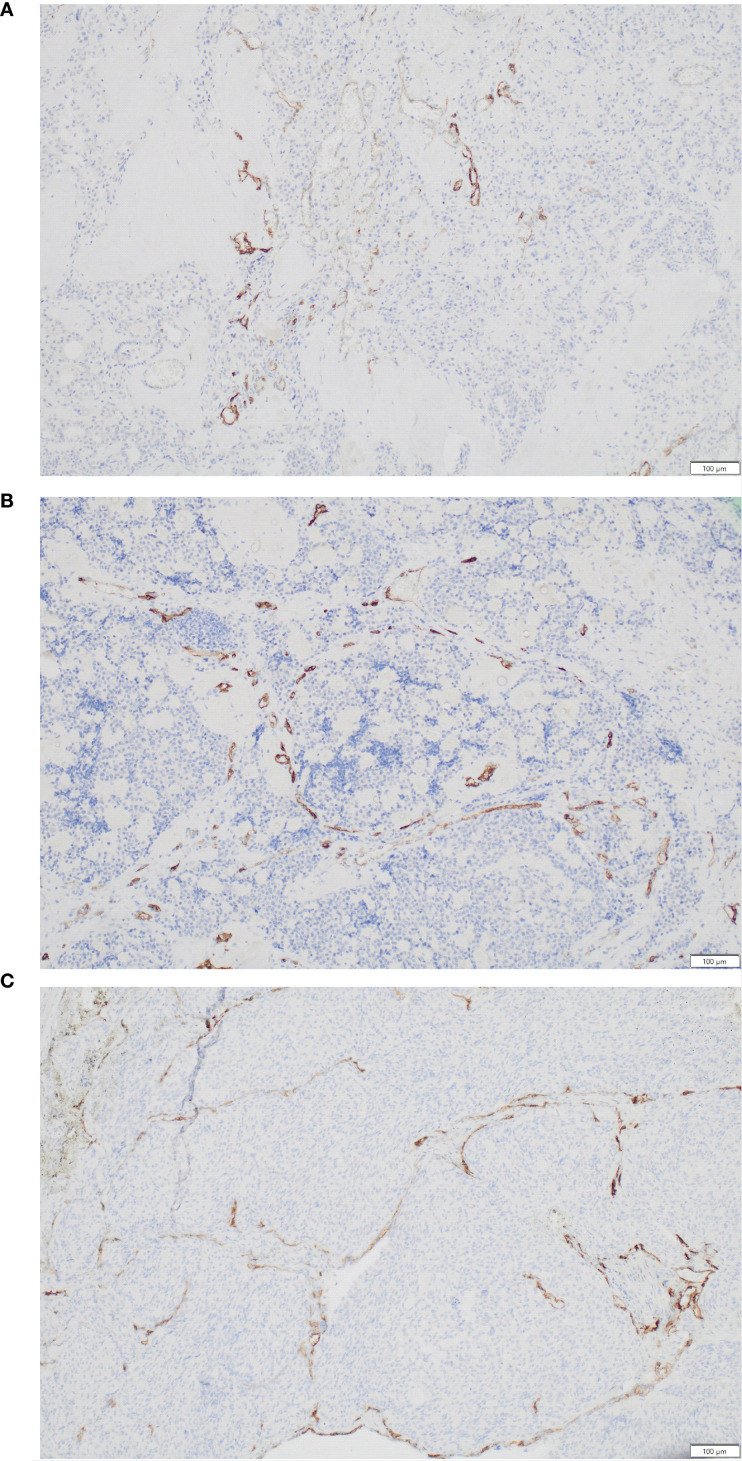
Different patterns of PSMA expression by medullary thyroid carcinoma. (A) PSMA expression by 10% of vessels (score 1). (B) PSMA expression by 15% of vessels (score 1). (C) PSMA expression by 55% of vessels (score 2).
